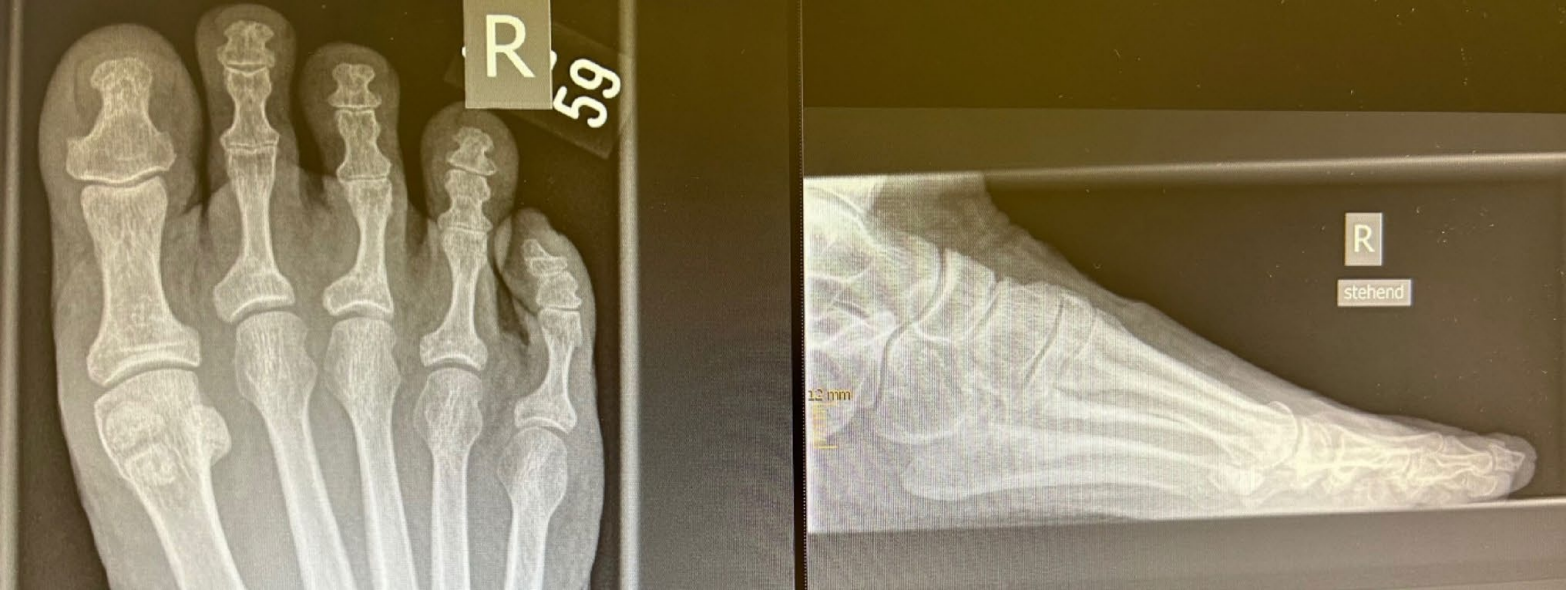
X-ray: long-term results after MIS
